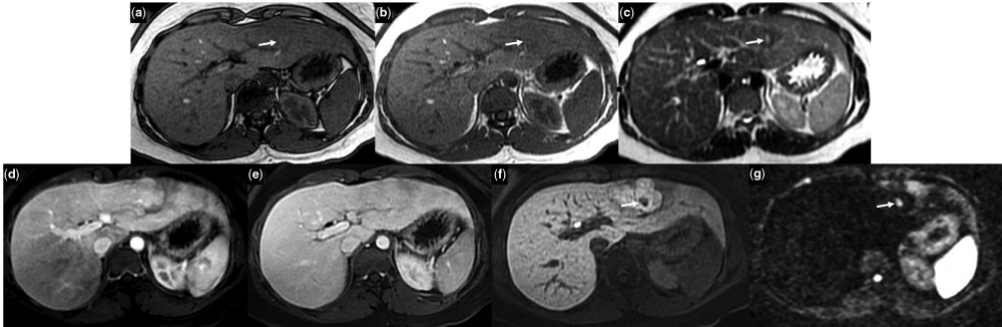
Magnetic resonance imaging demonstrating a nodule measuring 1.1 cm with smooth margins within a 6-cm mass with typical imaging features of focal nodular hyperplasia. This nodule presented the absence of signal dropout on opposed-phase T1-weighted image (arrow in a) in contrast to the in-phase T1-weighted image (arrow in b), strong hyperintensity on T2-weighted image differently from the adjacent lesion (arrow in c), hyperintensity in the arterial phase equal to the adjacent lesion (d), and no washout of contrast media in the venous phase in which it appeared isointense to the adjacent lesion (e). The nodule demonstrated strong hypointensity in the hepatobiliary phase (arrow in f) and strong restriction on diffusion-weighted image (arrow in g) compared with the adjacent lesion. These magnetic resonance findings were strongly suggestive for inflammatory hepatocellular adenoma.
